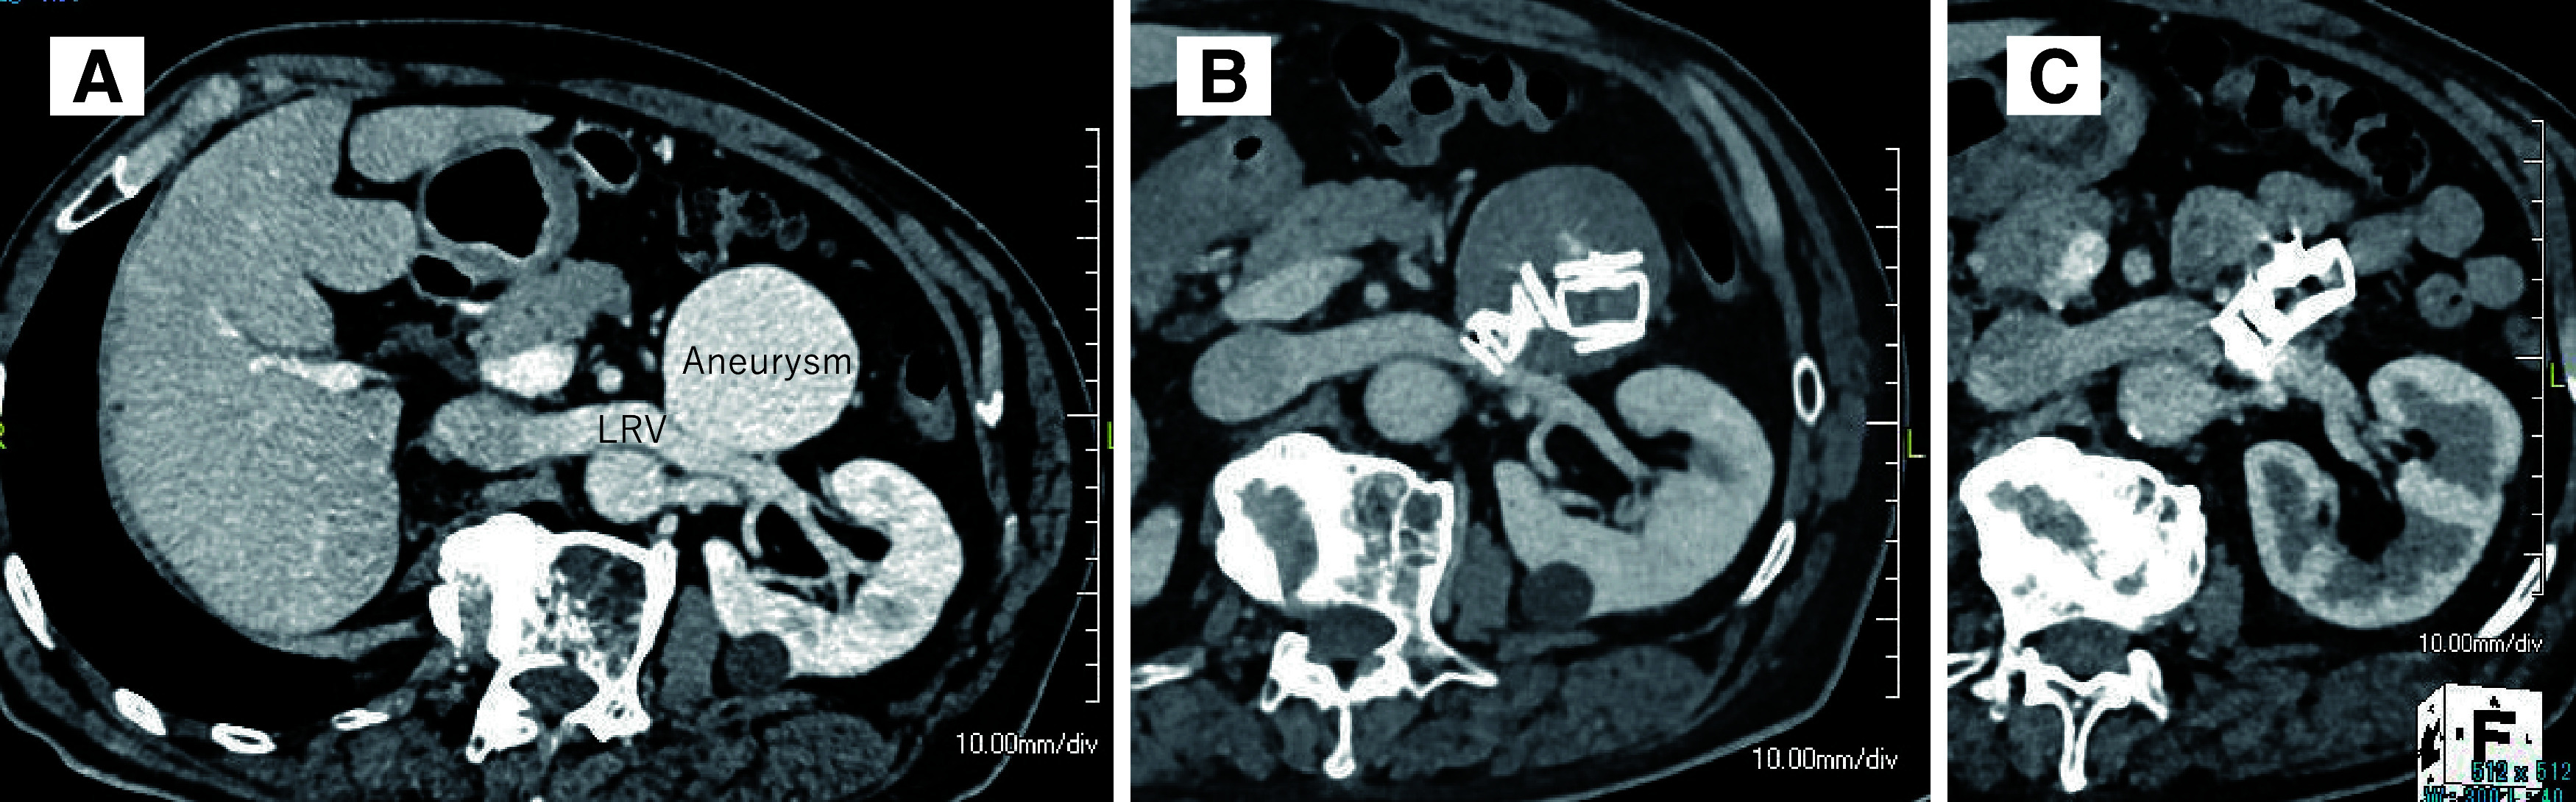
Fig. 1 Serial enhanced CT. (A) CT just before the intervention shows a large aneurysm, 53 mm in diameter, deriving from the LRV. (B) Immediately after the procedure. The left renal vein is patent. (C) One year after the procedure. The aneurysm has disappeared completely. No thrombus is present in the LRV. CT: computed tomography; LRV: left renal vein.
